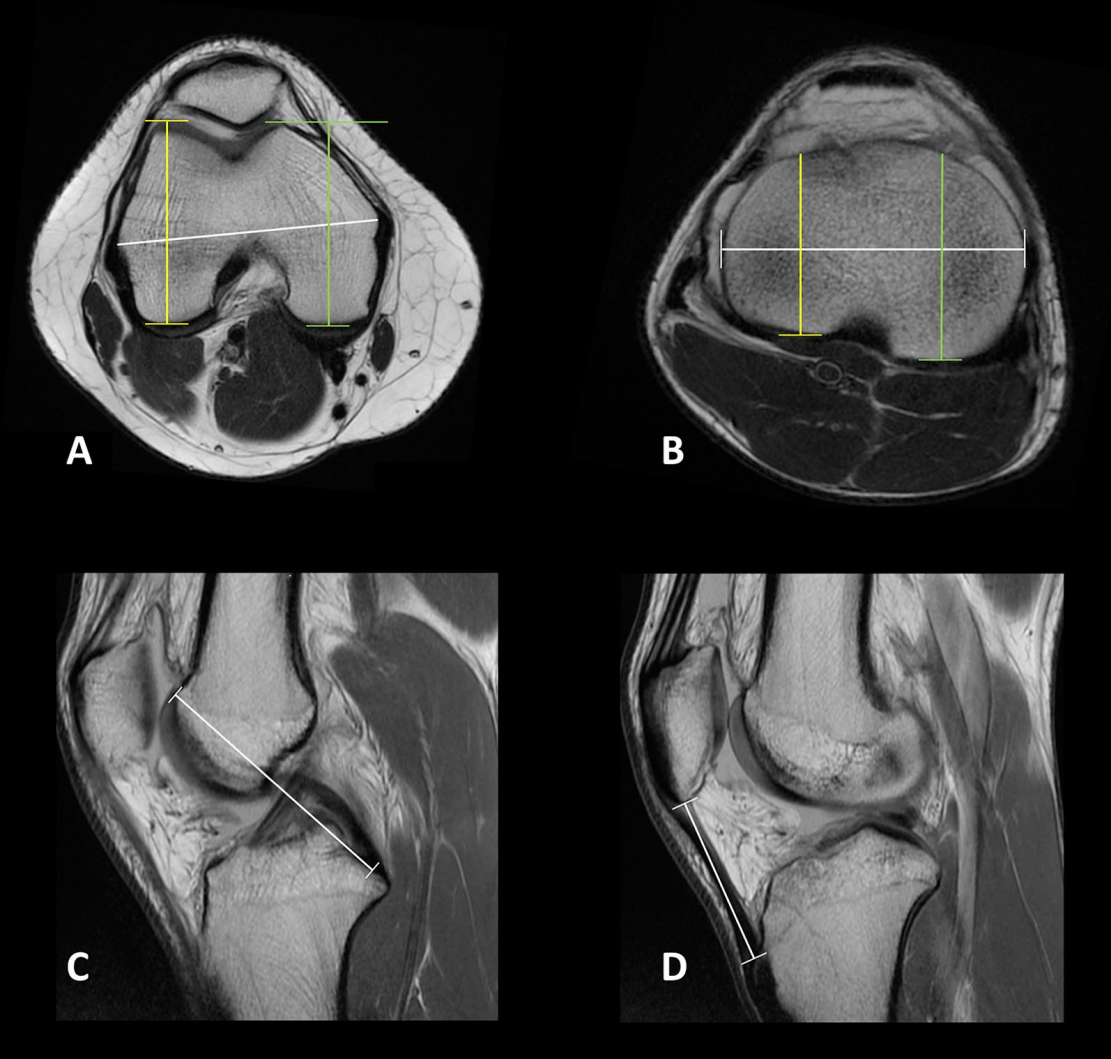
2D measurements on T1-weighted MRI sequences: A Axial view of the femur: transepicondylar Distance (TD, white line), medial femoral condyle anteroposterior (AP) dimension (MFAP, green line), and lateral femoral condyle AP dimension (LFAP, yellow line), measured on axial cut. B Axial view of the tibia: proximal tibia medio-lateral dimension (PTML, white line), medial tibial plateau AP dimension (MTAP, green line), lateral tibial plateau AP dimension (LTAP, yellow line). C Sagittal view: posterior cruciate ligament and trochlear cartilage distance (PCLTC, white line). D Sagittal view: patellar tendon length (PTL, white line)
